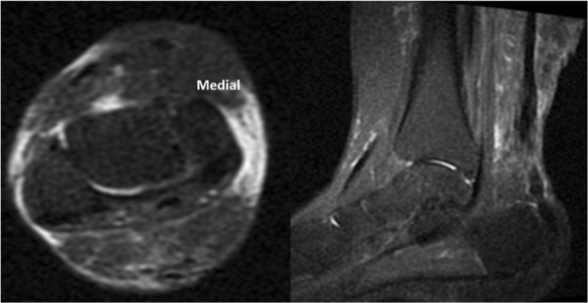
MR images obtained 28 days after surgery
